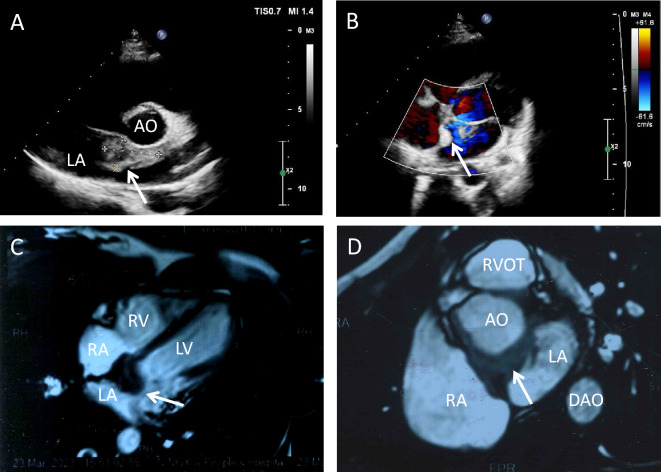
A Echocardiography and color Doppler blood flow image (B) from left atrium angle, white arrow indicates the tumor. C Nuclei magnetic resonance image from long axis, white arrow indicates the tumor. D Nuclei magnetic resonance image from aortic valve plane. White arrow indicates the tumor. *LA* left atrium, *AO* aortic, *RA* right atrium, *LV* left ventricle, *RV* right ventricle, *DAO* descending aorta, *LCS* left coronary sinus, *RCS* right coronary sinus, *NCS* left coronary sinus
